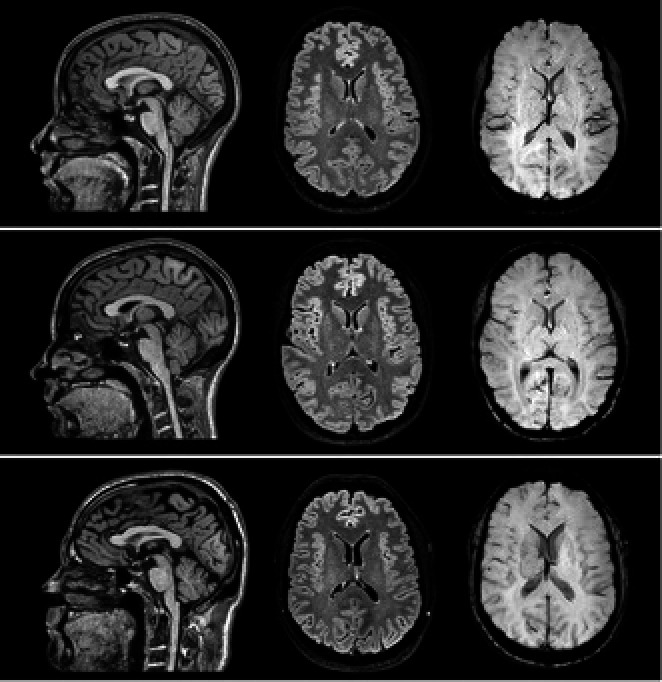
FIGURE 1 Brain MRI scans of the three subjects harboring the heterozygous CP gene variant: the proband, the older sister, and the father, from top to bottom. From left to right are shown a volumetric T1‐weighted, a T2‐weighted FLAIR and an SWI sequences.
